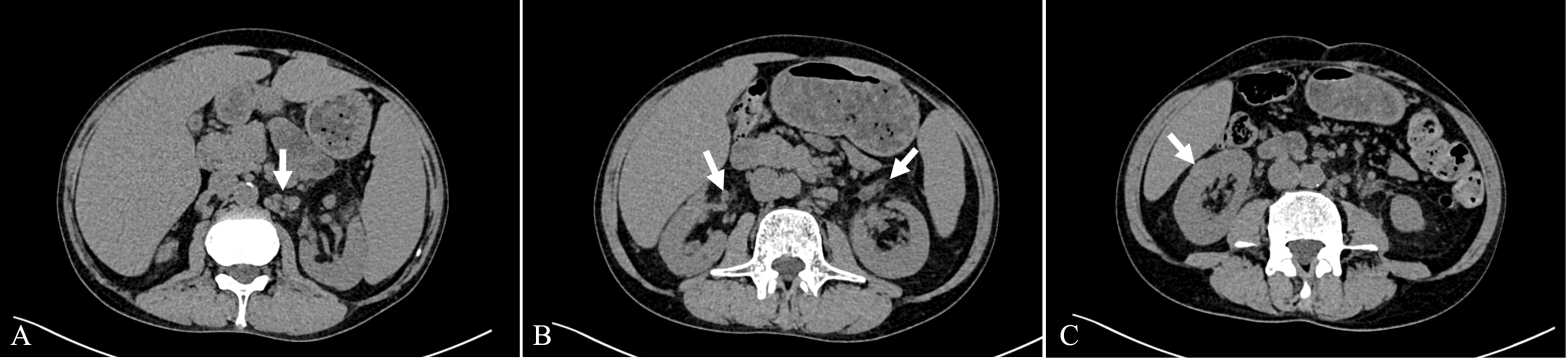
Axial view of the CT scan. (A) Enlarged lymph nodes in the retroperitoneal region. (B) Exudation around the renal pelvis. (C) Isodense nodular lesion under the renal capsule.
